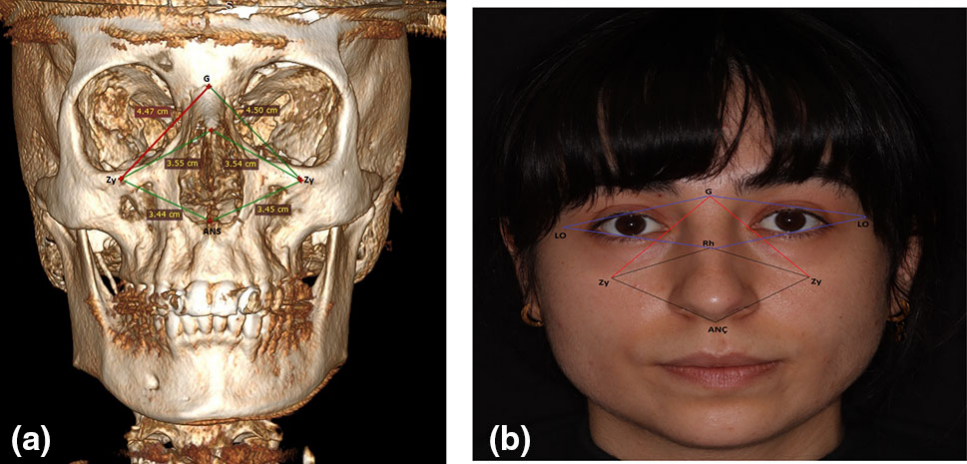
The measurement of distances between Zy-ANS, Z-Rh, Zy-G, G-LO, and Rh-LO on both sides of the face were depicted through 3D CT (a) and photography (b). Zy: Zygion, ANS: Anterior Nasal Spine, Rh: Rhinion, G: Glabella, LO: Lateral Orbit
